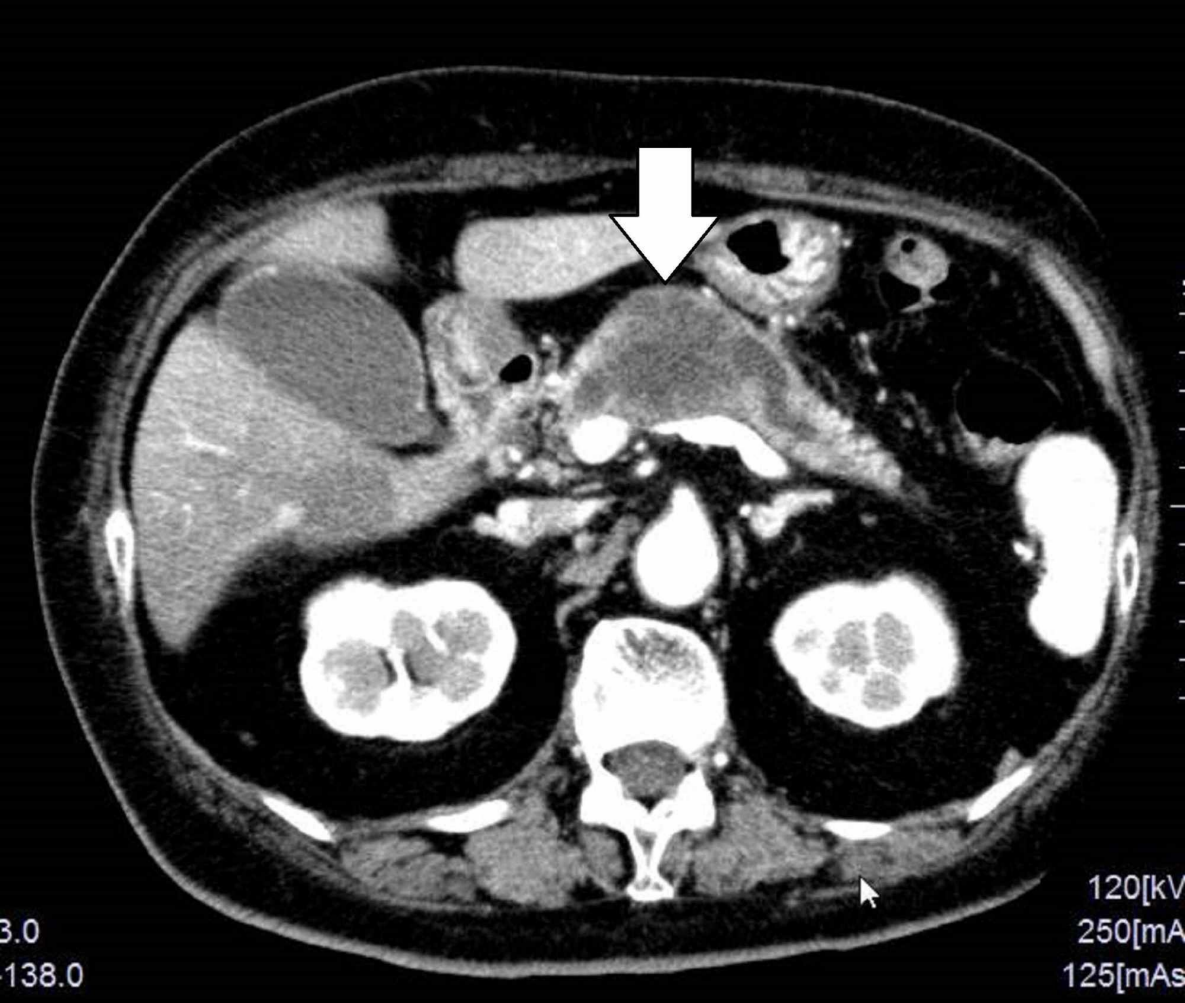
Dynamic enhanced computed tomography Dynamic enhanced computed tomography revealed a low density tumor in the body of the pancreas measuring 4 cm in diameter, with a poor contrast effect and unclear outline. The main pancreatic duct was dilated on both the head and caudal side of the tumor.
